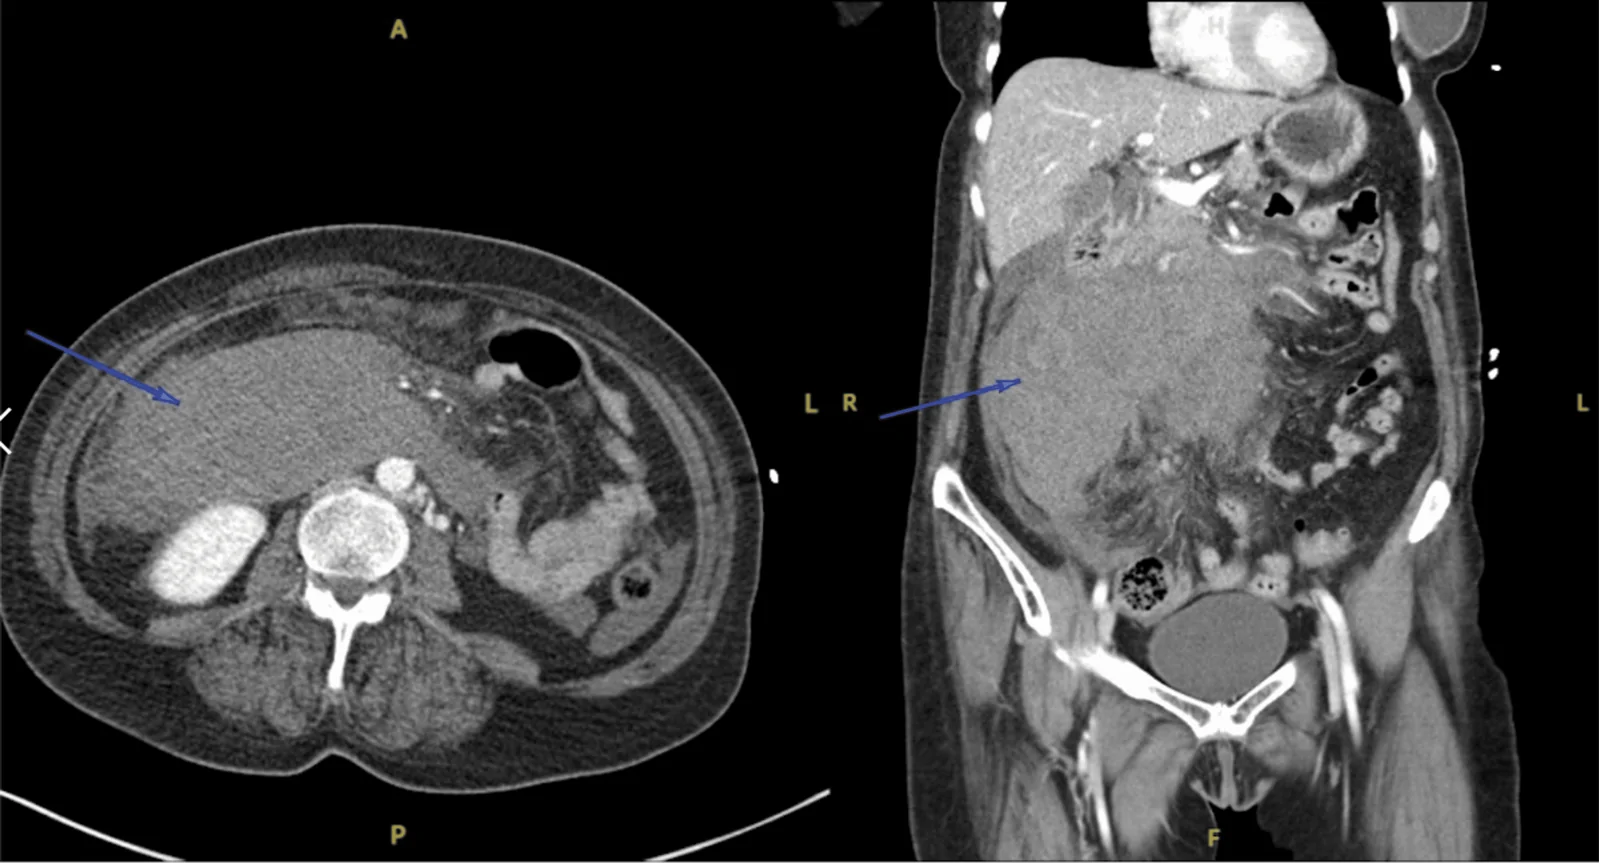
Initial contrast-enhanced CT demonstrated a large right hemiabdominal hematoma/fluid collection with surrounding inflammatory stranding and regional mass effect. The blue arrows point to a large mass-like structure in the right hemiabdomen with marked inflammatory stranding, likely a large fluid collection in the coronal view (left) and axial view (right).
